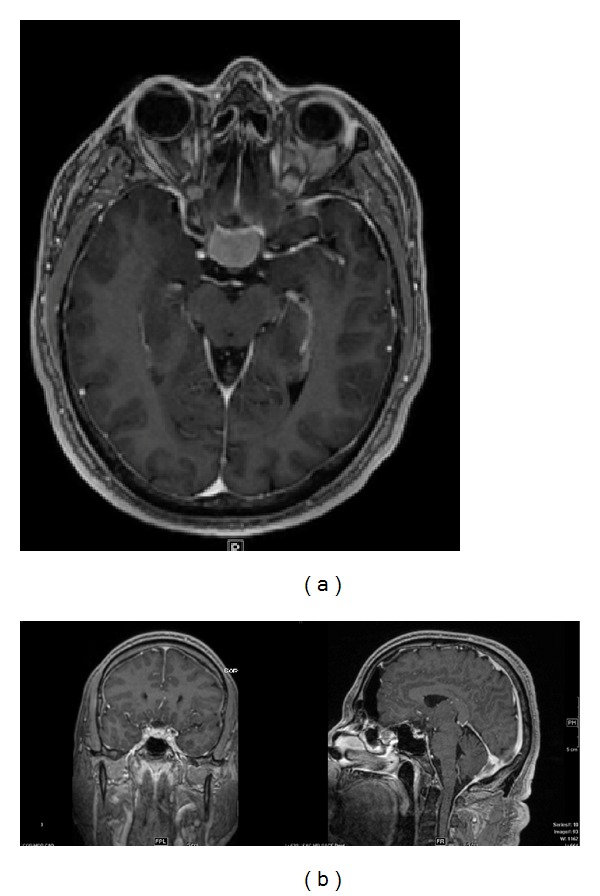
(a) Preoperative and (b) postoperative MR images of a homogenously enhancing mass involving the planum sphenoidale. Pathology was meningioma. Gross total resection was achieved.
